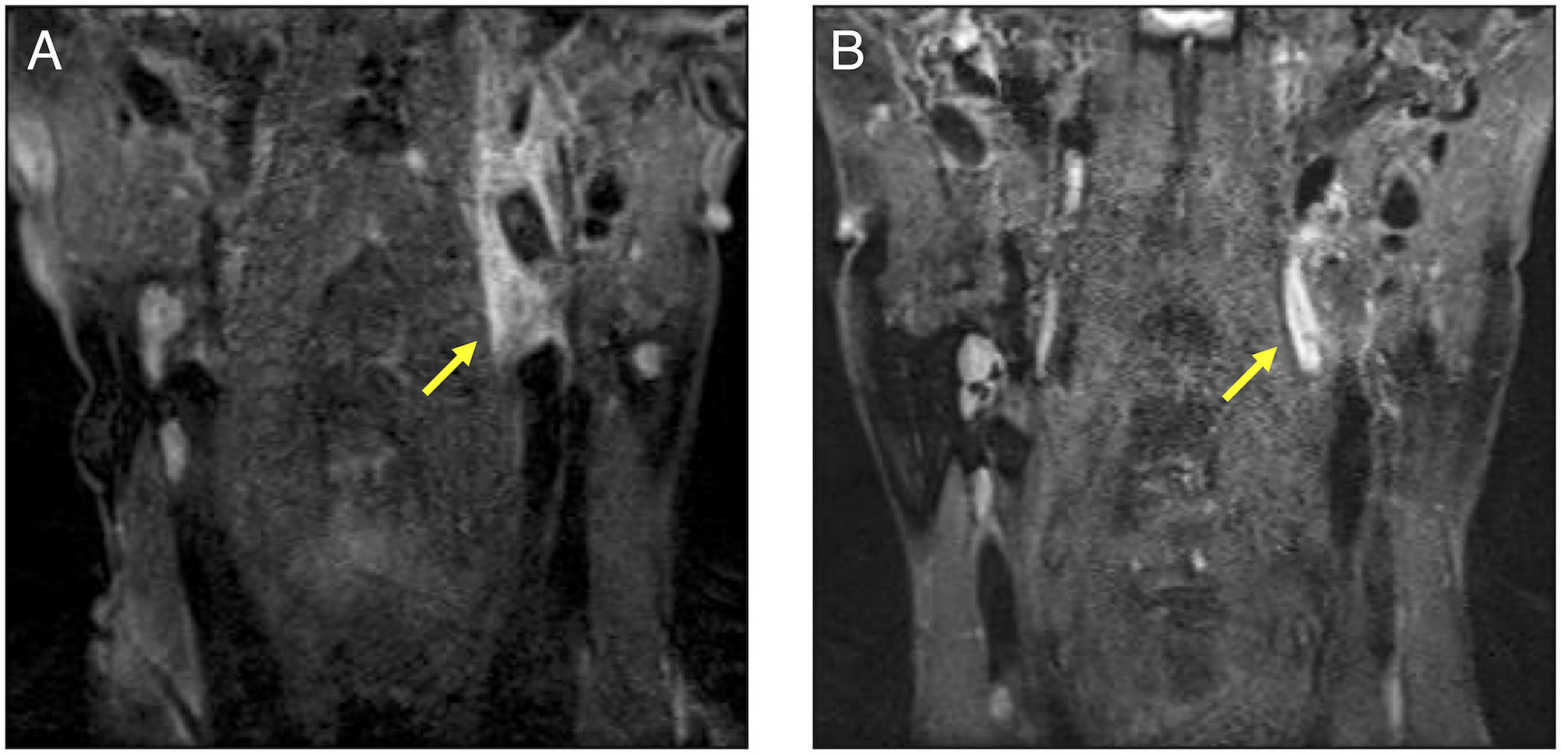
A cervical contrast-enhanced MRI comparison before and after the treatment in our patient. (A): This MRI was performed before treatment. Aneurysm, stenosis, and dissections were observed in the left internal carotid artery indicated by the arrow. A contrast effect was also observed around the lesion. (B): The participant underwent MRI as an outpatient after treatment. The contrast effect around the left internal carotid artery had decreased. MRI, magnetic resonance imaging.
